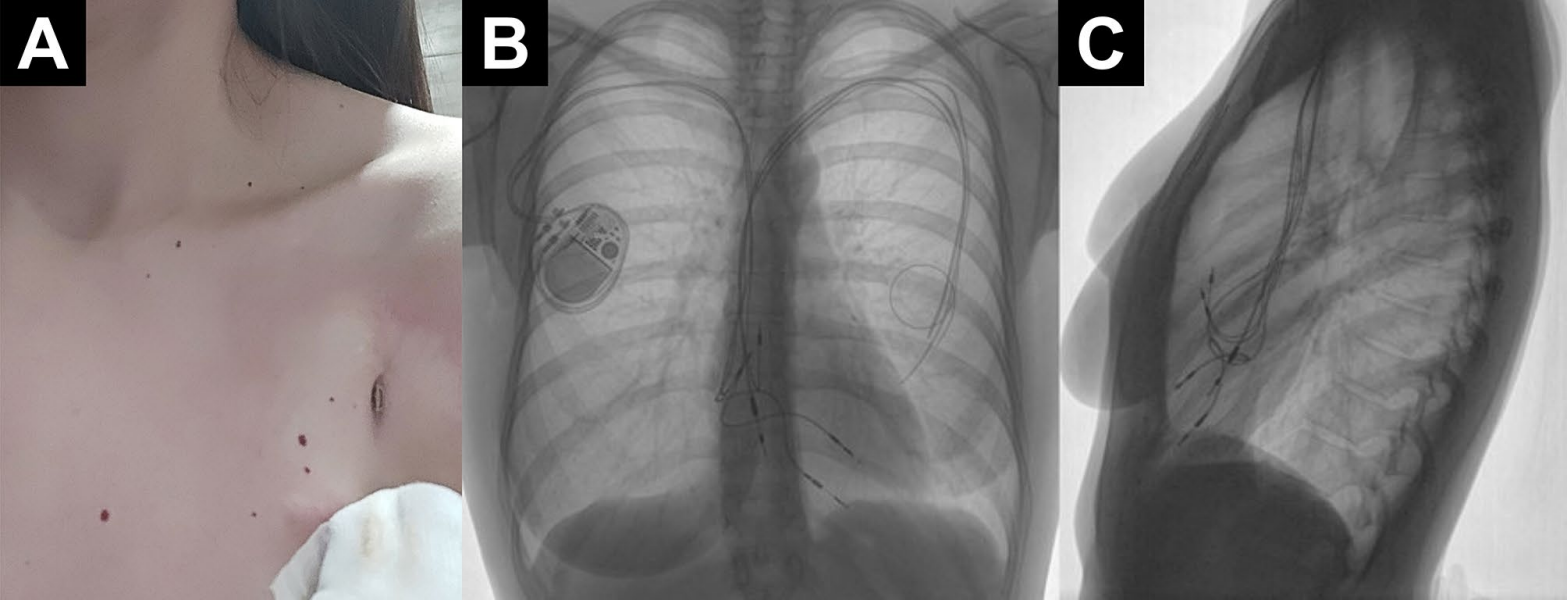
Photograph and chest x-ray of case at 16 months postpartum. Photograph of individual in case study at 16 months postpartum showing a chronic skin fistula above the left-sided pocket (A), and antero-posterior (B) and lateral (C) view of chest x-ray made before the retained leads extraction. Retained left-sided leads and completely functional right-sided pacemaker system are clearly visible on the chest x-ray
